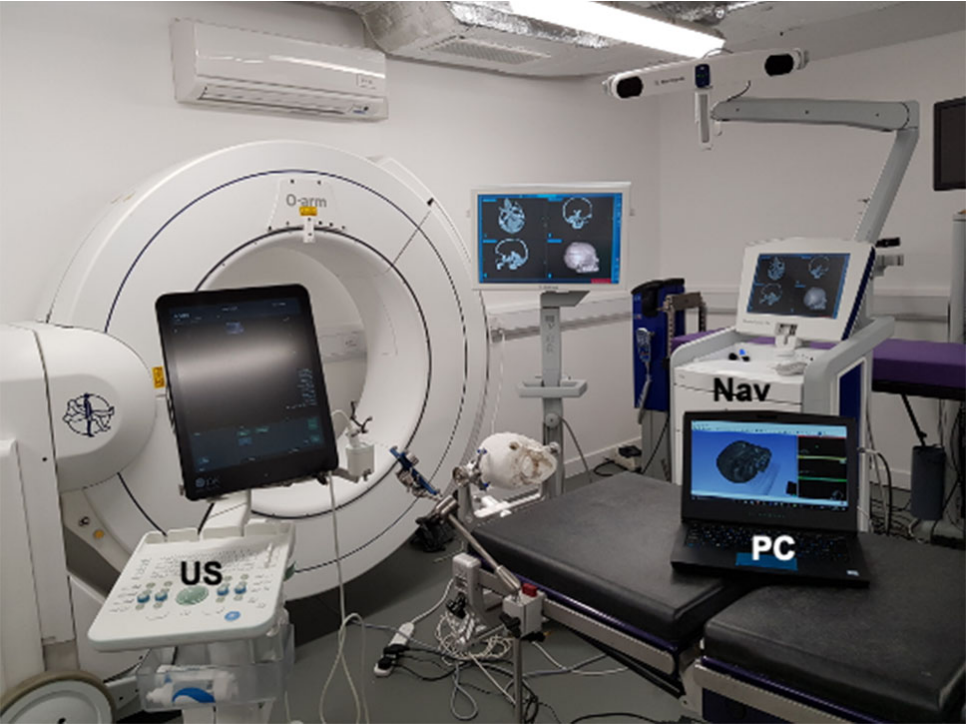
System setup—Nav: Medtronic Stealthstation, US: BK 5000 Ultrasound system, PC: Laptop
